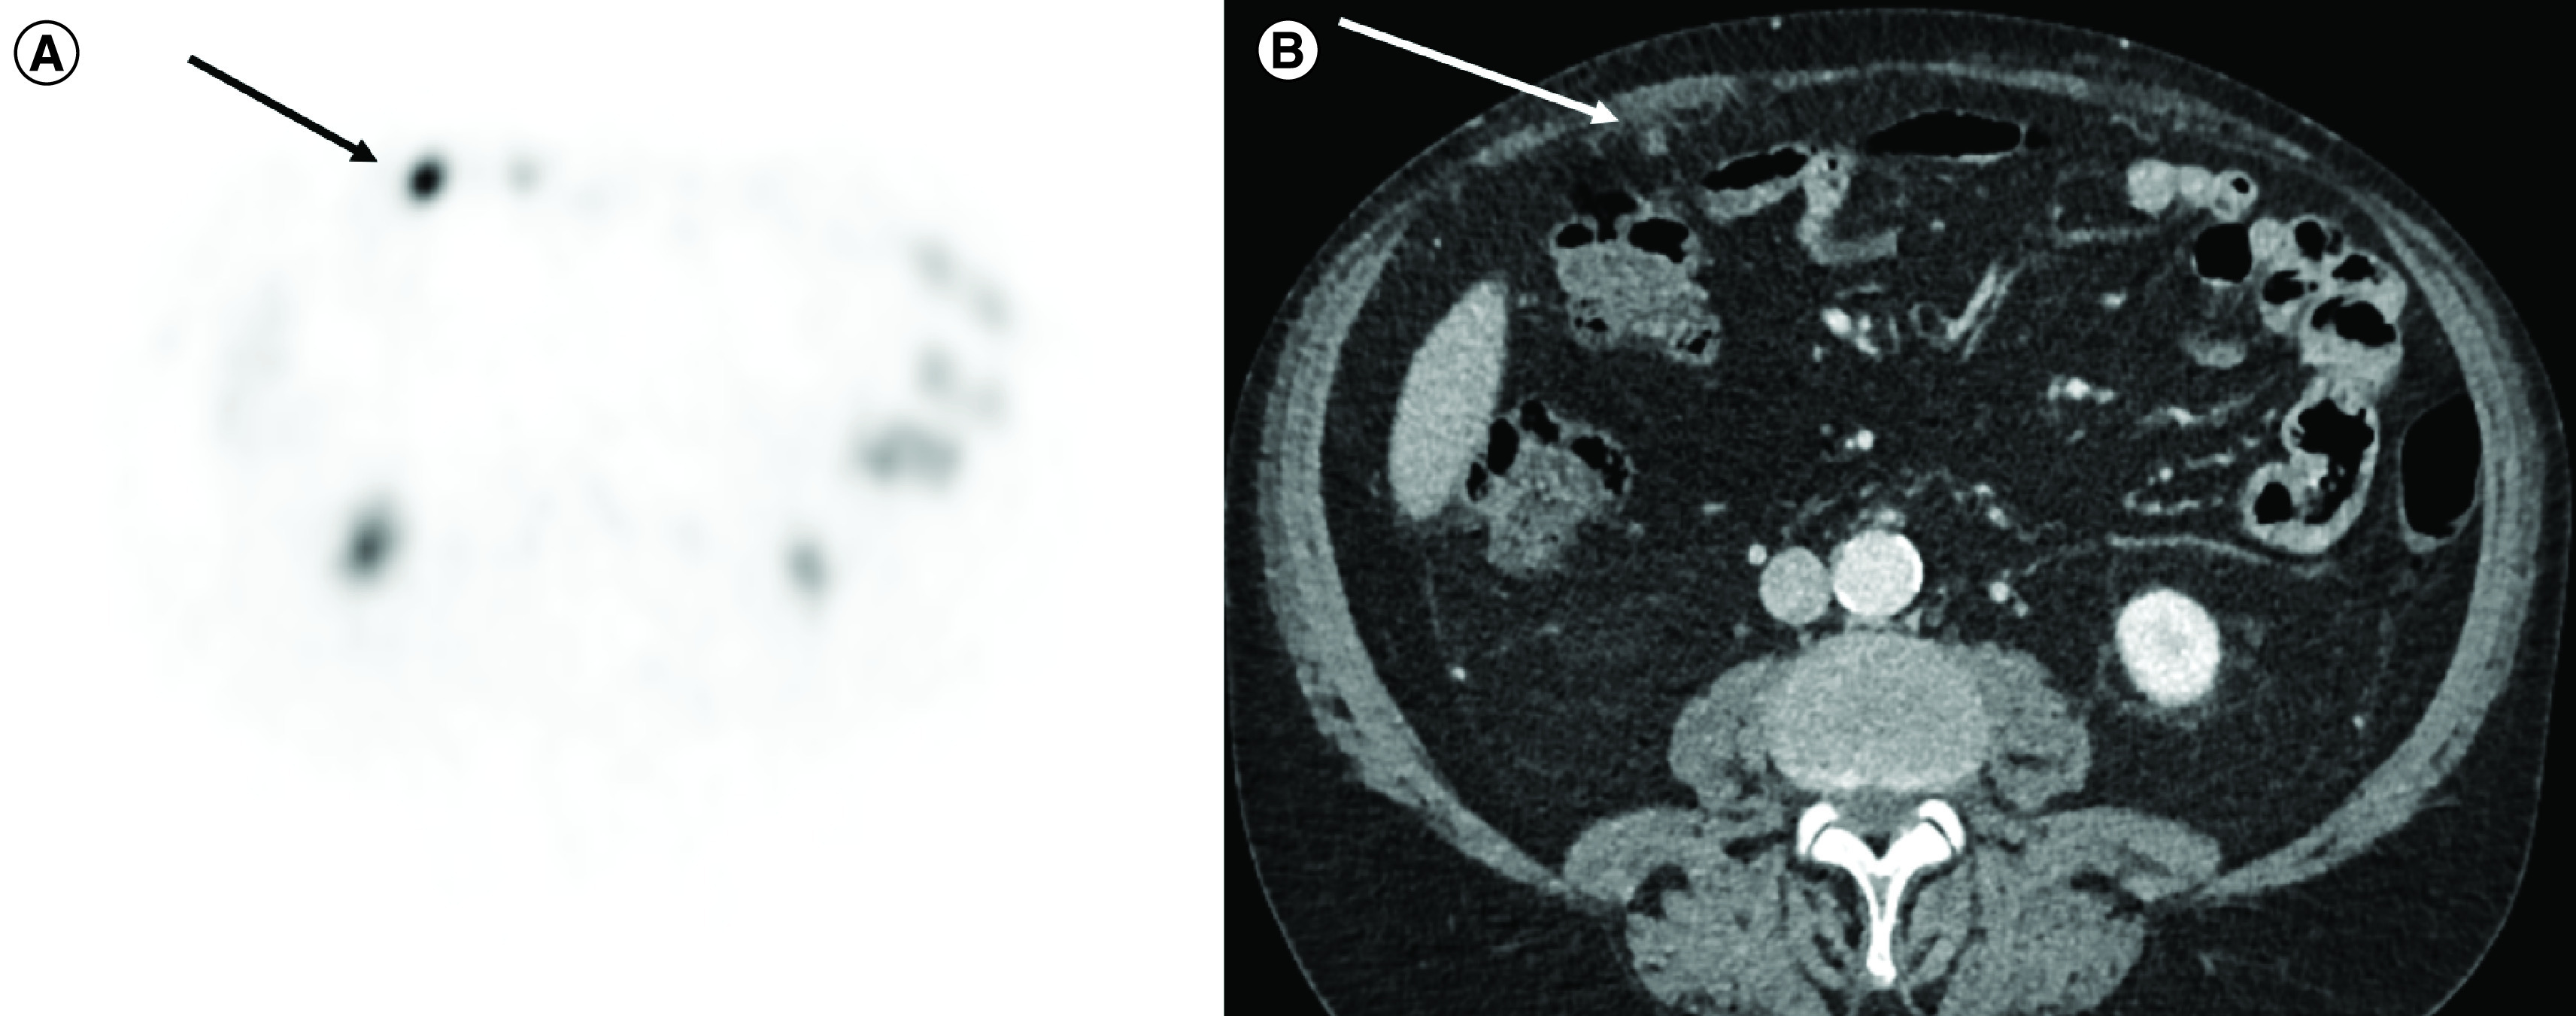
(A) PSMA-PET showing peritoneal implant (arrow). (B) Abdominal computed tomography that was performed on the same time and that initially misdiagnosed these peritoneal implants due to its very small size (arrow).
